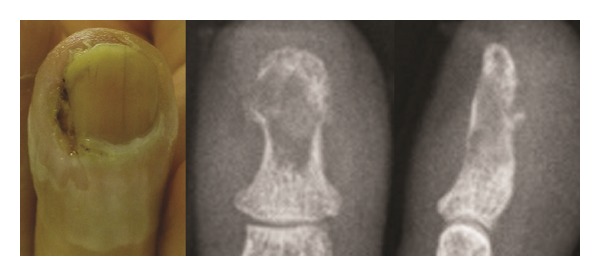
Anteroposterior and lateral radiographs at the first presentation.
